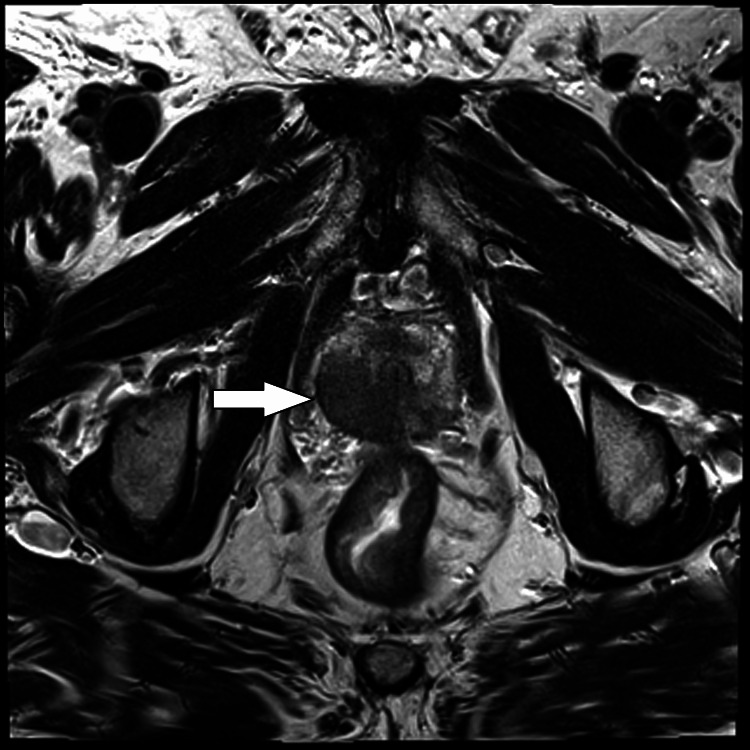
Multiparametric magnetic resonance imaging of the prostate: 30mm PI-RADS 5 lesion with characteristic T2 hypointensity involving the right peripheral zone and transition zone from base to apex.
